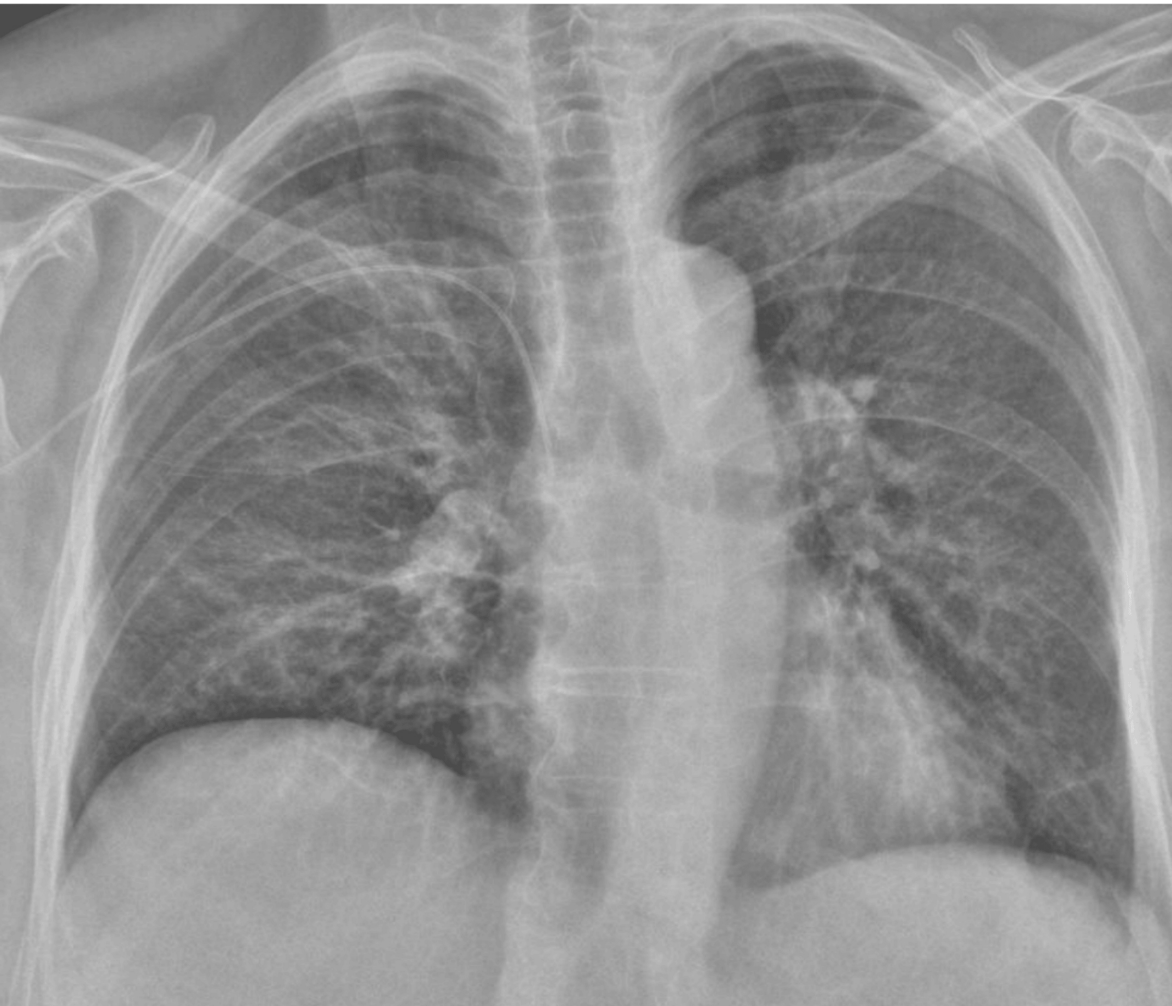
Chest radiograph showing radiological improvement of ground-glass changes after treatment with monoclonal antibody, antivirals, and immunoglobulins.
